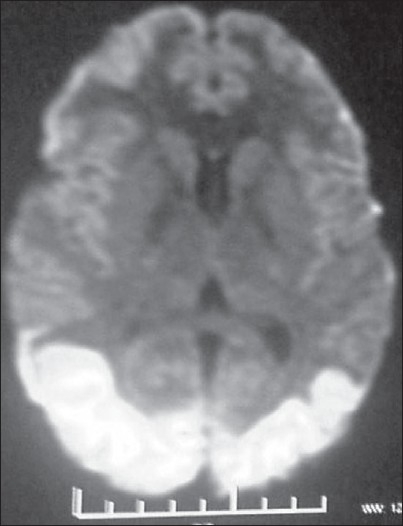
Diffusion-weighted MRI of the brain of the second patient showing hyperintensities in the bilateral occipital lobe
